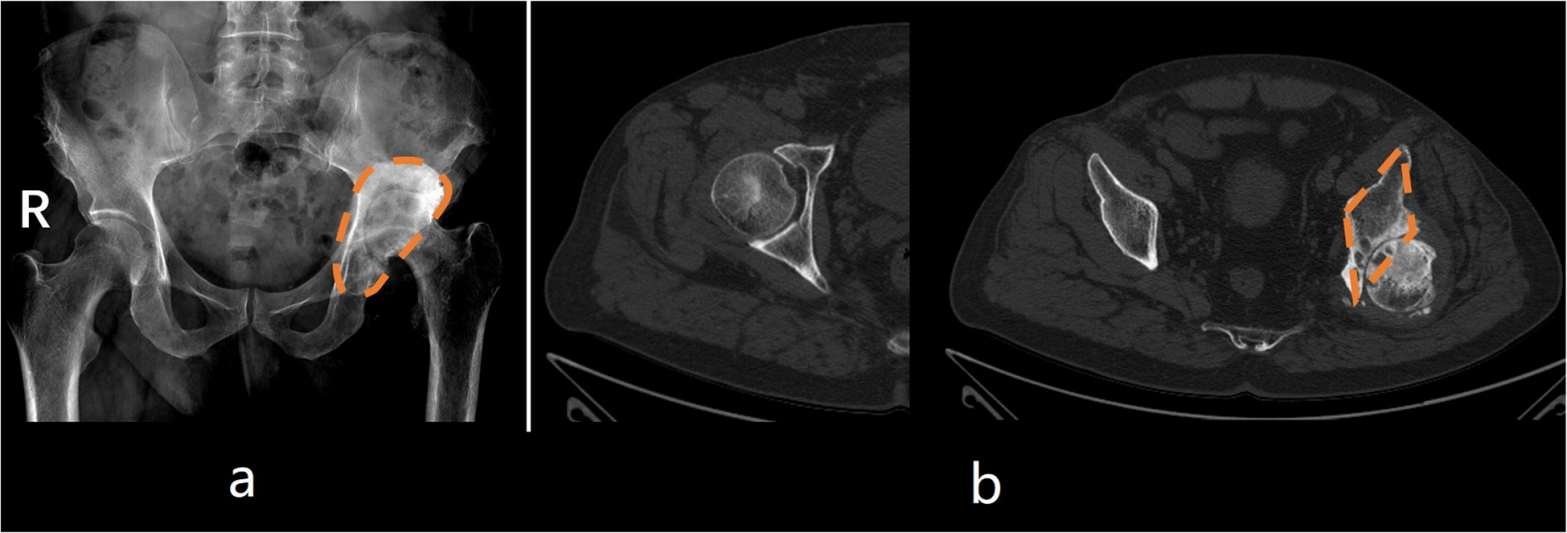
Radiological characteristics of patients with long-term unreduced hip joint dislocation, secondary osteoarthritis and pseudoarthrosis. a On normal anterior-posterior view X-ray examination, a semicircular high-density area could be identified in typical cases. Because the area looks like the lunar aureole (pseudoacetabulum) around the moon (femoral head), it is called the “aureole” sign. When an aureole sign is identified together with a slightly upward shift of the rotation center of the hip joint, the diagnosis of long-term unreduced hip joint dislocation, secondary osteoarthritis and pseudoarthrosis must be considered to avoid the possibility of misdiagnosis. There is no objective quantitative index to describe the aureole sign. We estimate that the aureole sign can be observed in 13/16 patients (this is based on subjective results obtained by one observer). b. On axial CT, when the rotation center is identified, the anterior and posterior column and the internal wall of the acetabulum resemble a large letter “I” in healthy people. However, in patients with long-term unreduced hip joint dislocation, secondary osteoarthritis and pseudoarthrosis, the ilium, dome of the original acetabulum and the internal/posterior wall of the pseudoacetabulum will form a rhombus, which is called the “rhombus” sign. This sign might be helpful in avoiding the possibility of misdiagnosis. There is no objective quantitative index to describe the rhombus sign. We estimate that the rhombus sign can be observed in 11/16 patients (this is based on subjective results obtained by one observer)
